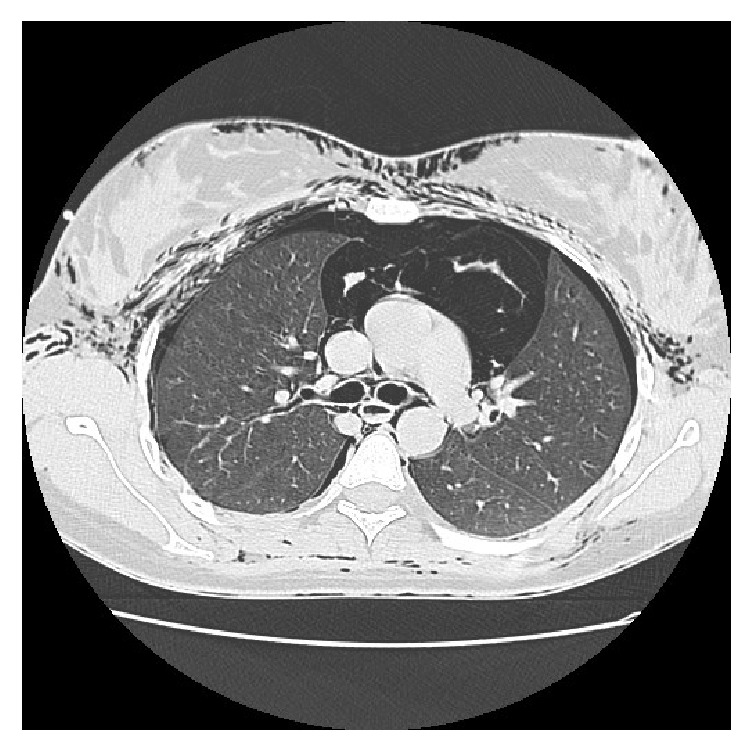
Thoracic computed tomography showing pneumomediastinum, subcutaneous emphysema, and minimal pneumothorax.
